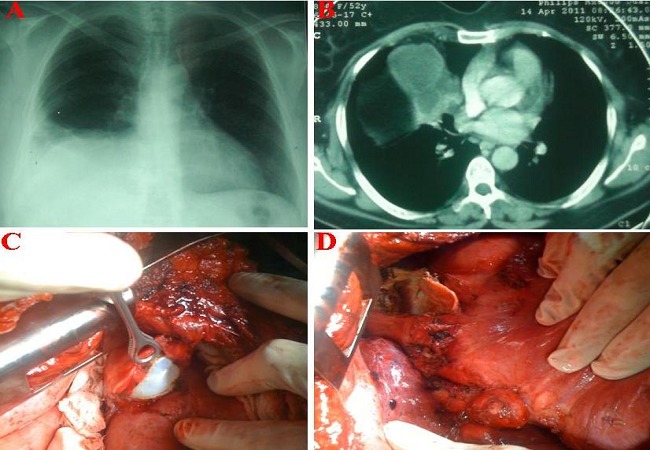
A-Chest X-ray showed large opacity of the inferior right hemithorax with elevation of diaphragm, B-Chest-CT showed cystic lesion in the diaphragm area, C-Peroperative view confirming the diaphragmatic location of the hydatid cyst, D-Peroperative view showed multiple hydatid cyst of the diaphragm
